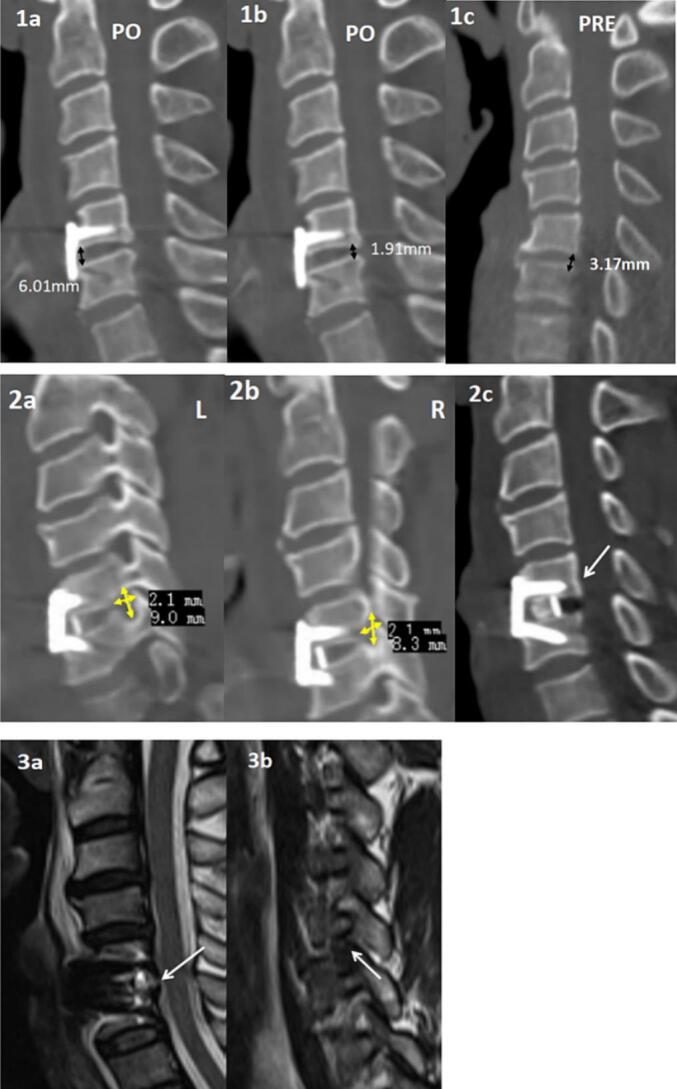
(1a-2c) Postoperative CT:(1a) Anterior disc space height postoperatively(1b) Posterior disc space height postoperatively(1c) Preoperative posterior disc space height(2a) Postoperative left foramen height and width(2b) Postoperative right foramen height and width(2c) White arrow indicating mild posterior displacement of C5 vertebra(3a-b) Postoperative MRI:(3a) White arrow indicating thorough decompression of the spinal cord(3b) White arrow indicating stenosis of the right foramen.
